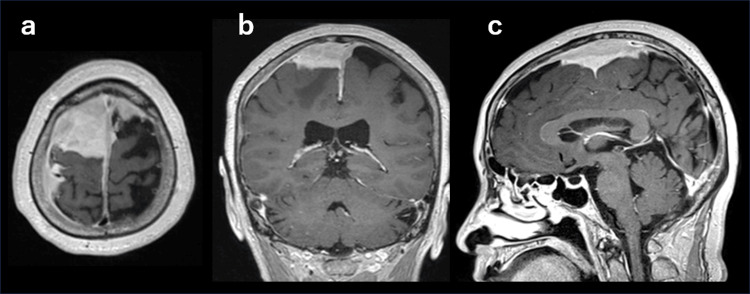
Gadolinium-enhanced magnetic resonance imaging. (a) Axial view. (b) Coronal view. (c) Sagittal view.
